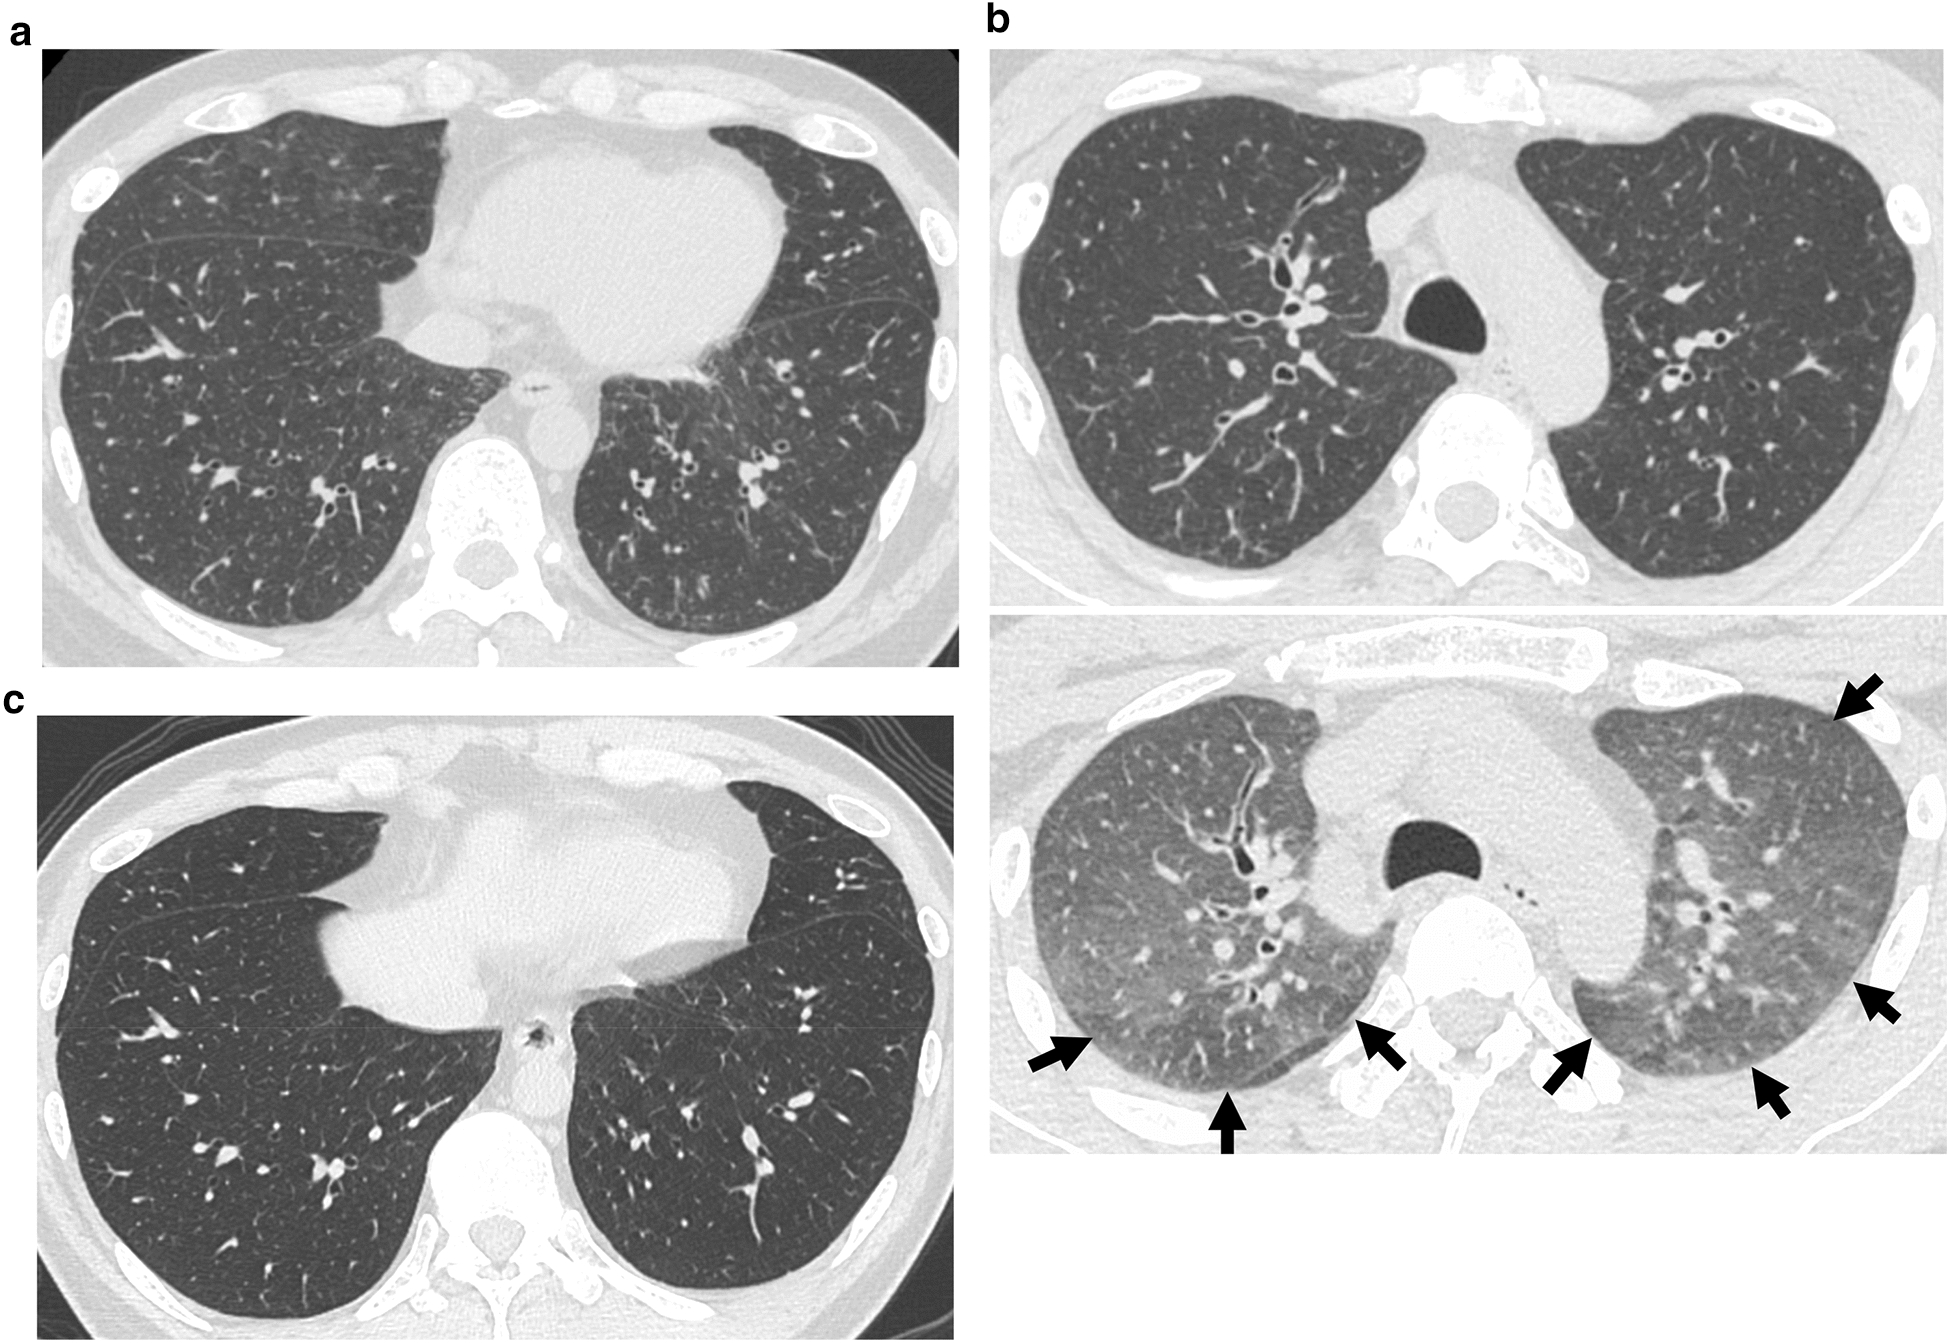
Axial HRCT image of the chest on admission (a, b) and post-ART (c). a Multiple centrilobular small nodules and branching opacities within all lung lobes, associated with small areas of ground-glass opacities (GGO) in the peribronchiolar region and bronchial wall thickening are seen. b Axial HRCT image on expiratory scan (lower image) shows lobular and subsegmental areas of mosaic pattern (arrowheads) due to air trapping in small airways, which is not evident from the inspiratory scan (upper image). c Improvement in interstitial abnormalities of the lung compared to pre-ART
